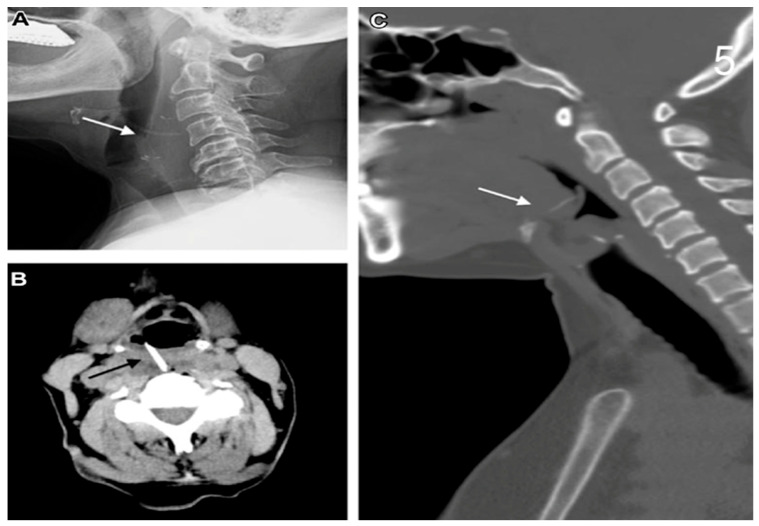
(A) Cervical X-Ray showing FB (chicken bone). (B) Cervical CT scan showing FB, hyperdense body. (C) CT cervical scan showing rectilinear body.
